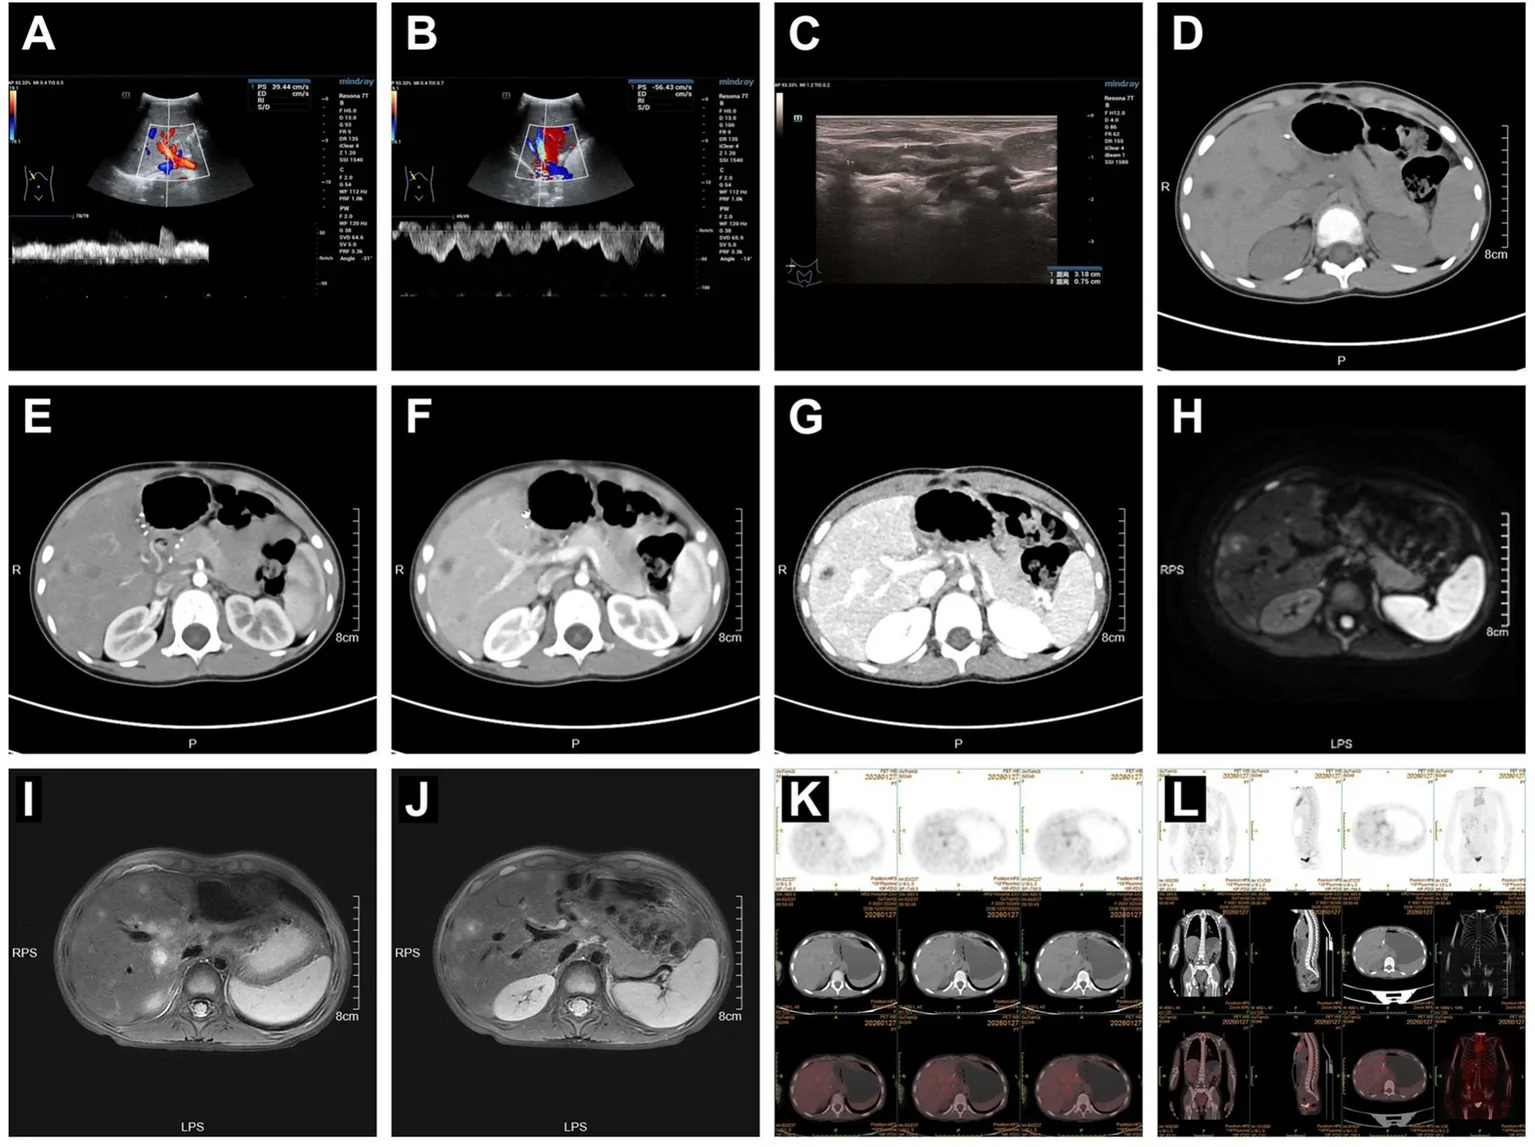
Pre-treatment imaging of allograft recurrence (12–27 January 2026). This imaging constitutes the recurrence work-up performed before salvage chemotherapy (which began in February 2026). (A–C) Ultrasonography on 12 January 2026 showed multiple cystic lesions in the liver allograft, some with wall thickening and internal echogenic nodules. (D–G) Contrast-enhanced CT on 22 January 2026 showed multiple round low-density hepatic lesions with transient perilesional and rim enhancement. (H–J) Enhanced MRI on 24 January 2026 showed multiple long T1/long T2 nodular lesions with partial DWI hyperintensity and peripheral enhancement. (K, L) PET-CT on 27 January 2026 showed heterogeneous increased hepatic glucose metabolism, supporting suspected allograft recurrence. Post-chemotherapy follow-up imaging (February–April 2026) is described in the text.
